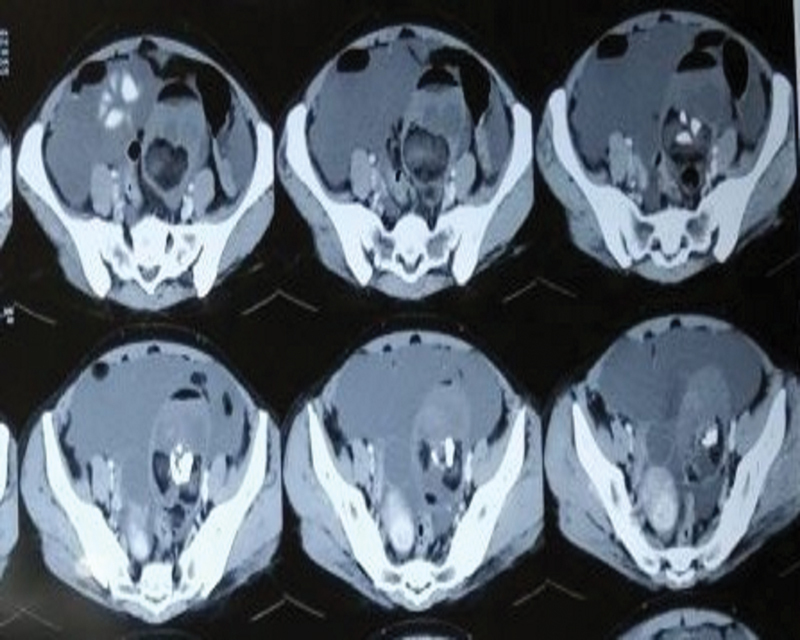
Contrast-enhanced computed tomography (CT) scan image of abdomen and pelvis (axial view) depicting left ovarian teratoma.
